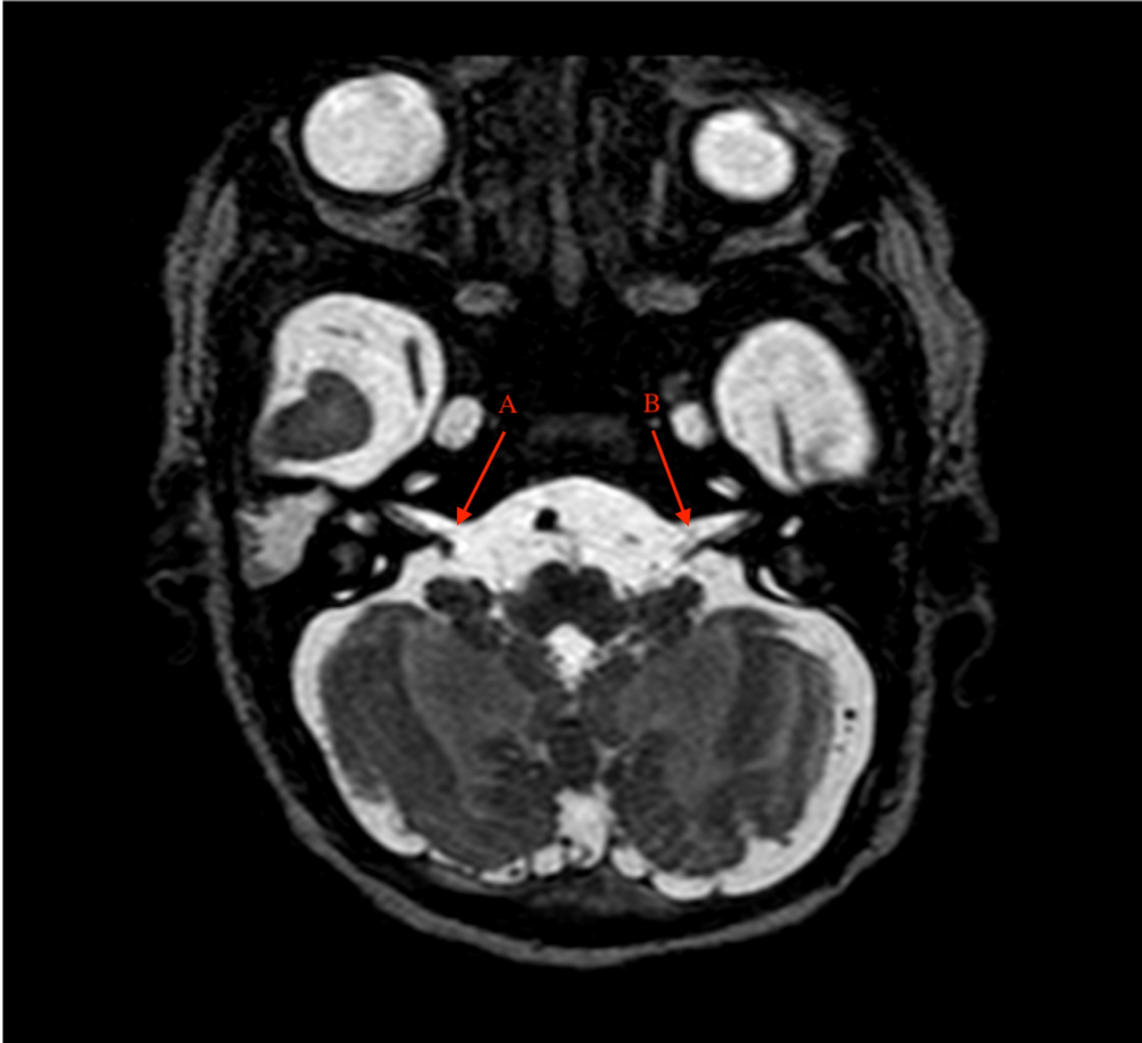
MRI brain showing absent right facial nerve (A) and hypoplastic left facial nerve (B).
